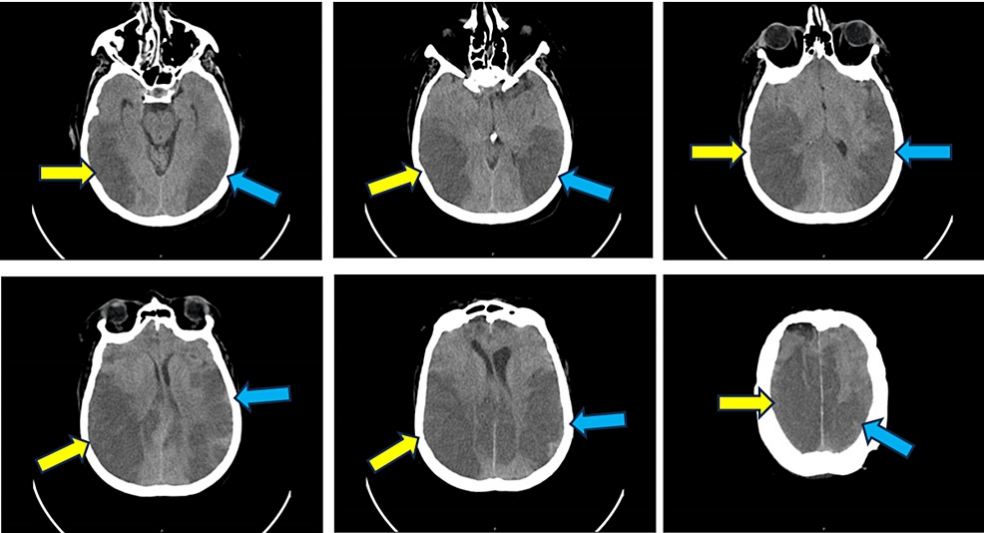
CT scan of a bilateral stroke with ascending cuts of the ischemic area in the right hemisphere (yellow arrow) and left hemisphere (blue arrow)
